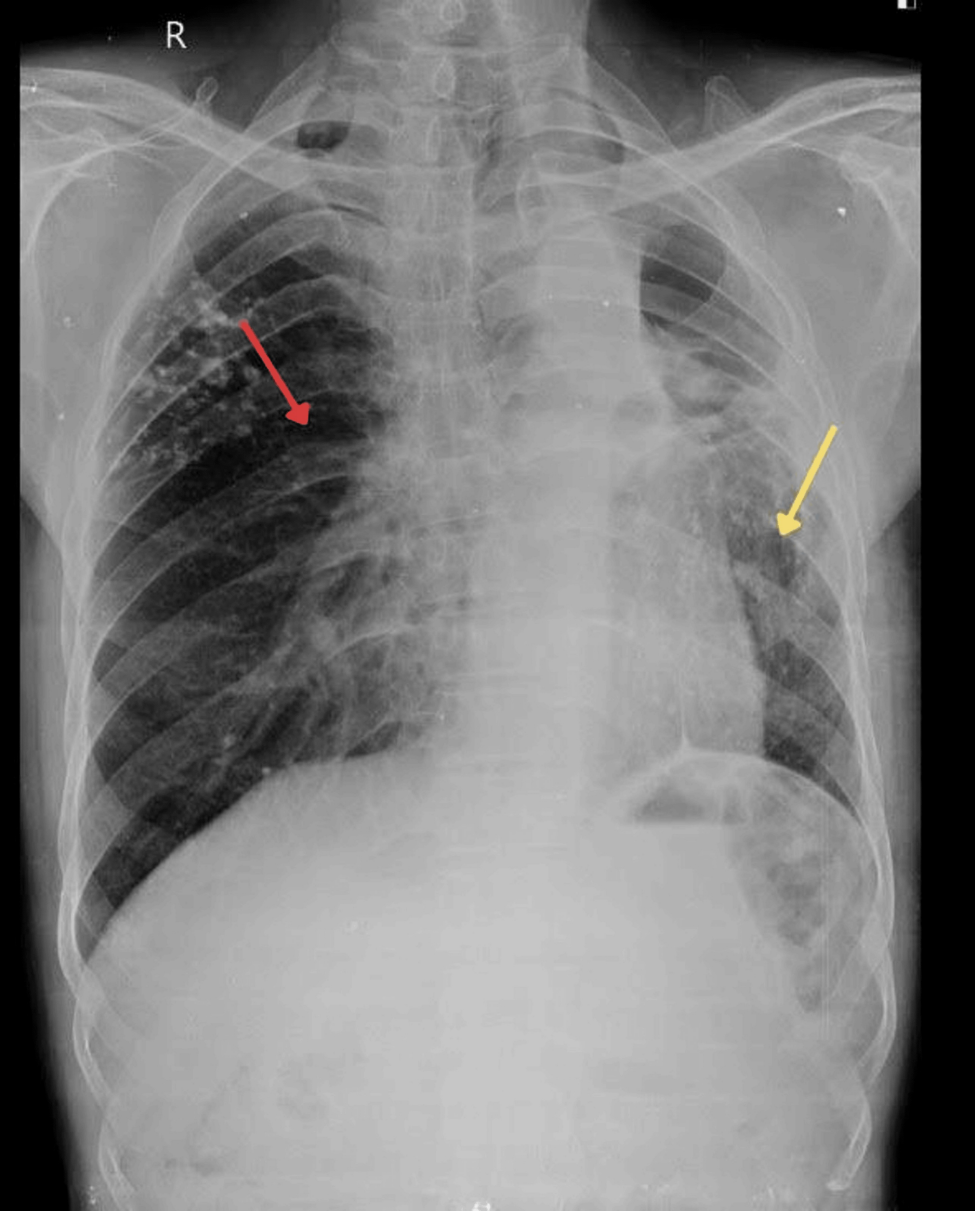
The X-ray AP view shows a destroyed left lung (yellow arrow) and a hyperinflated right lung (red arrow). AP: anterior posterior
